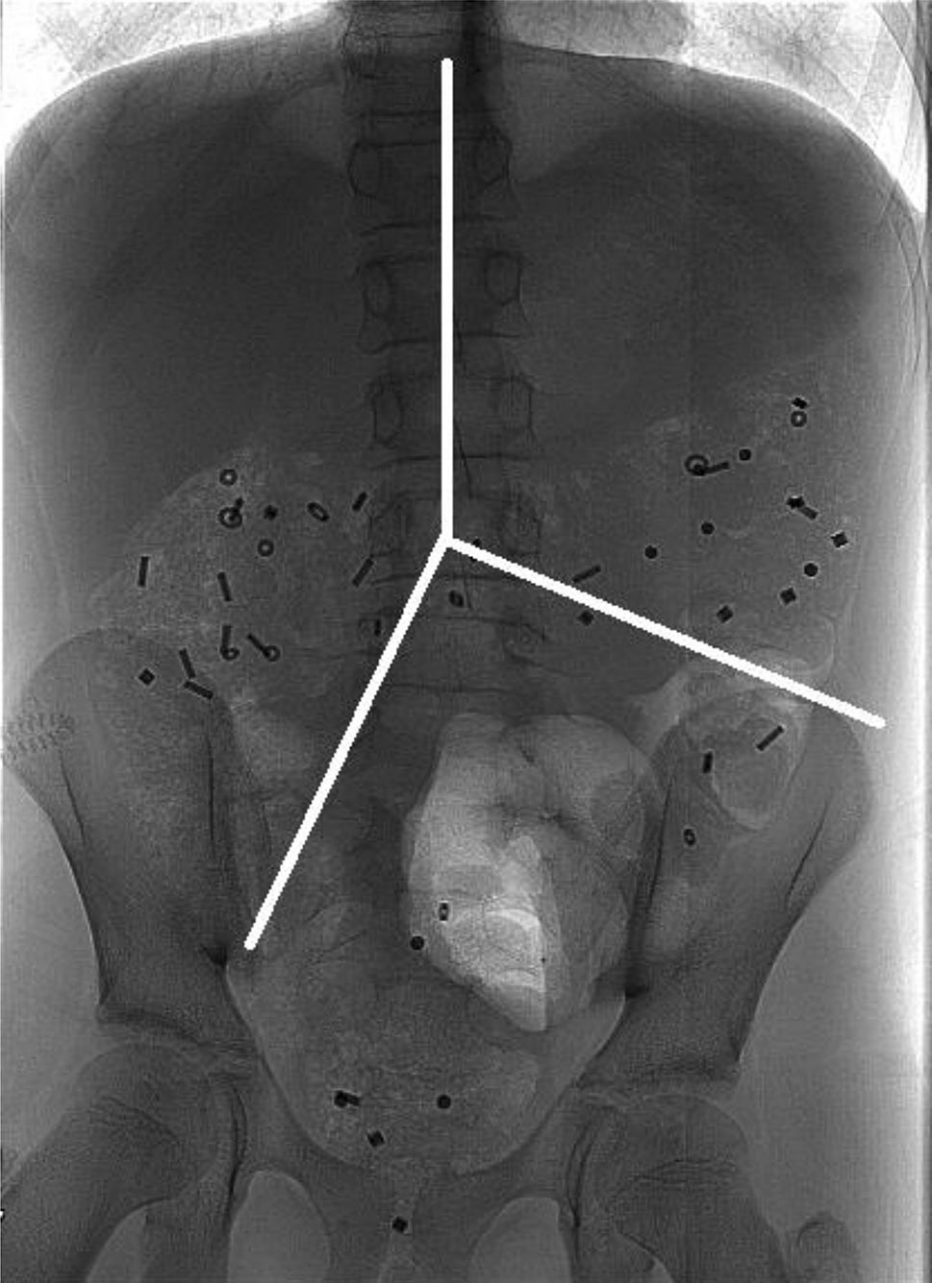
Abdominal frontal x-ray with colonic segments. In this frontal x-ray containing radio-opaque markers, the imaginary white lines mark the areas for segmental colon transit times. From the center of vertebrae L5, one line runs cranially along the vertebrae. The two other strait lines run towards the left iliac crest and the right pelvic outlet. The formed upper right area, the upper left and the lower left areas represents the right colon, the left colon and the recto-sigmoid colon respectively.
